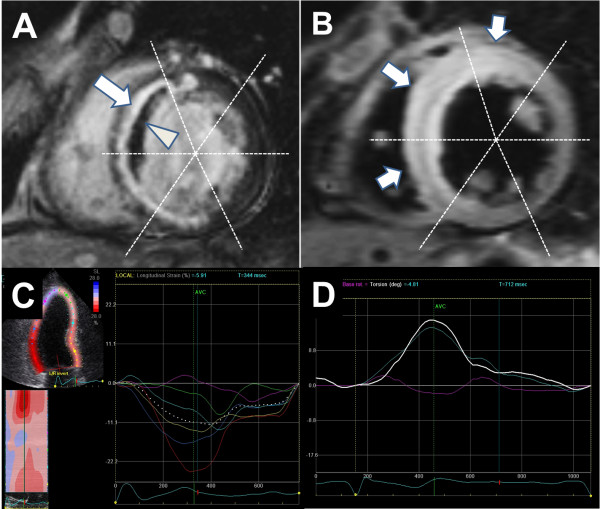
Measurement of extent of myocardial injury and myocardial function. Segmentation of left ventricle when measuring extent of myocardial injury from late gadolinium enhancement imaging (A) and T2 weighted imaging (B). Representative images of measuring global longitudinal strain (C) and twist (D) from speckle tracking echocardiography. Arrow in (A) indicates LGE and arrow head indicates microvascular obstruction. Arrows in (B) indicate higher signal intensity which represents myocardial edema.
